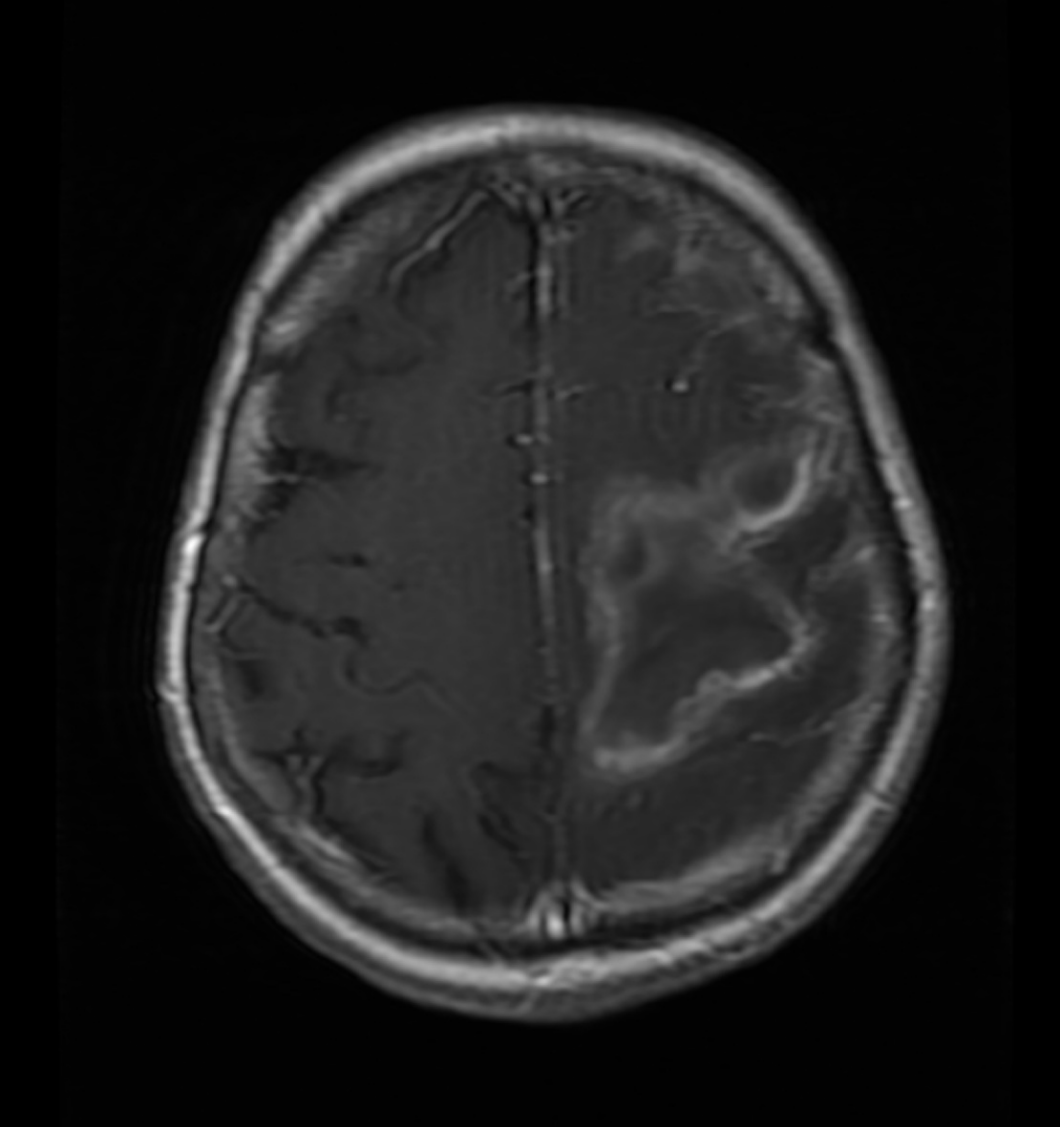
Axial postcontrast T1 weighted MRI showing worsening cerebral edema
